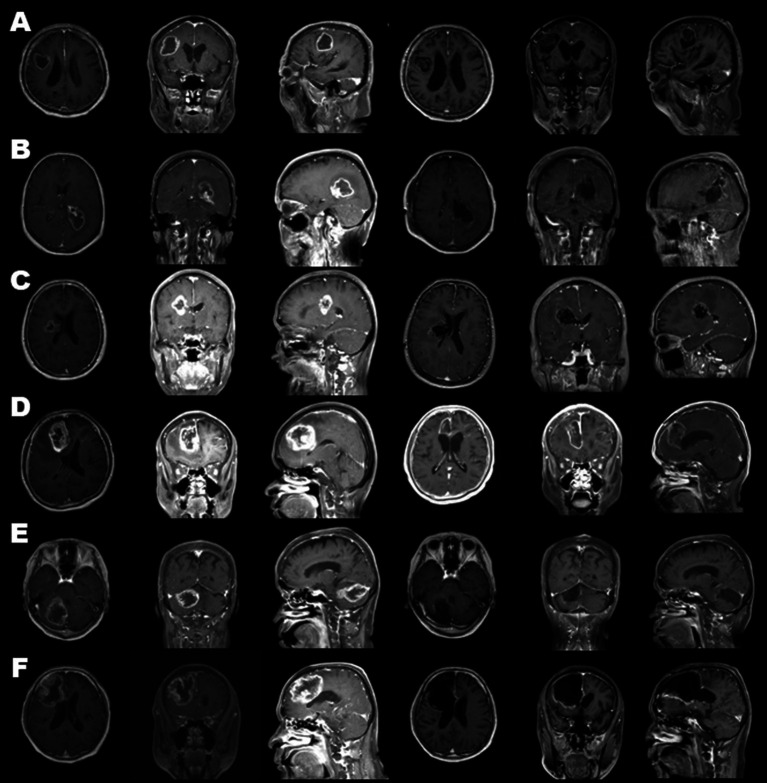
Preoperative (three columns on the left) and postoperative (three columns on the right) gadolinium-enhanced magnetic resonance images of all cases. Cases 1, 2, 3 (A–C) were treated through tumor removal only in an inside-out fashion. Cases 4, 5, 6 (D–F) combined excision in an outside-in fashion. As a result, gross-total resection in cases 1, 4, and 5 (A,D,E) and near-total resection in cases 2, 3, and 6 (B,C,F) was achieved.
